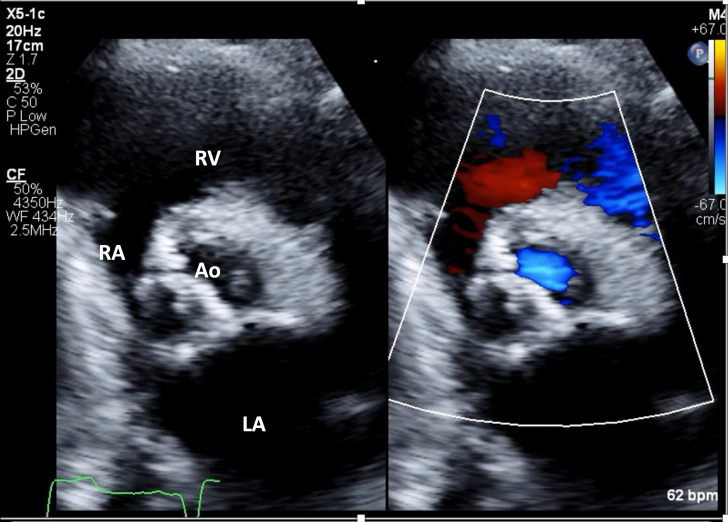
Short-Axis Evaluation of the Aortic Valve Parasternal short-axis view of the aortic valve used to interrogate valve morphology (identify bicuspid, tricuspid, etc) Note the location of calcium or presence of raphe. Abbreviations as in Figure 2.
